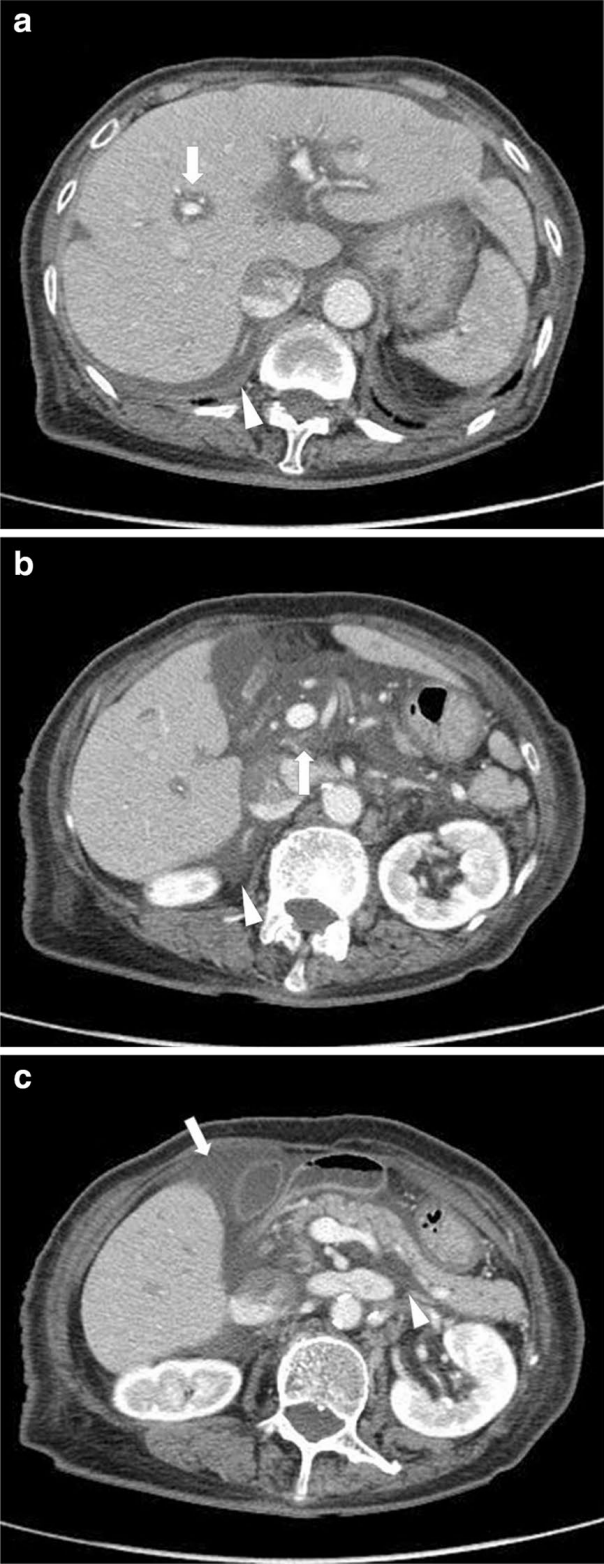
Axial contrast-enhanced computed tomography at the level of left portal vein of the liver (a), main portal vein (b), and pancreatic body (c). The periportal collar sign (*arrow*) and lymphedema in the bare area of the liver (*arrowhead*) are depicted in (a). Lymphedema in the hepatoduodenal ligament (*arrow*) and bare area of the liver (*arrowhead*) are depicted in (b). Subserosal edema of the gallbladder wall (*arrow*) and lymphedema in the anterior pararenal space (*arrowhead*) are depicted in (c).
